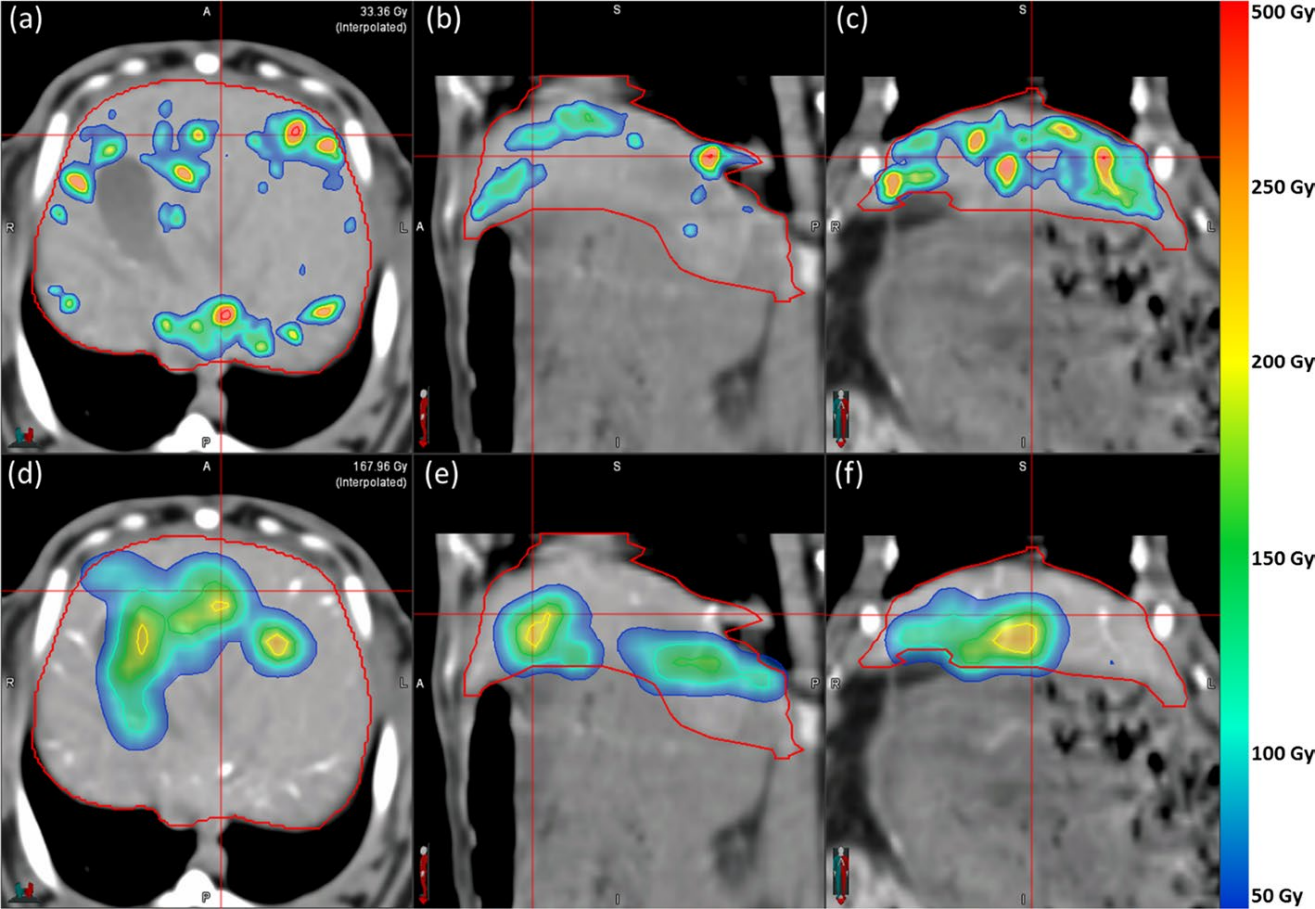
a–c Axial, sagittal, and coronal views of the CT-based dose distribution $${\text{DD}}_{{{\text{CT}}}}$$ in R05 overlaid on an axial CT [− 100 to 200 HU]. d–f Axial, sagittal, and coronal views of the PET-based dose distribution $${\text{DD}}_{{{\text{PET}}}}$$ in R05 overlaid on an axial CT [− 100 to 200 HU]
